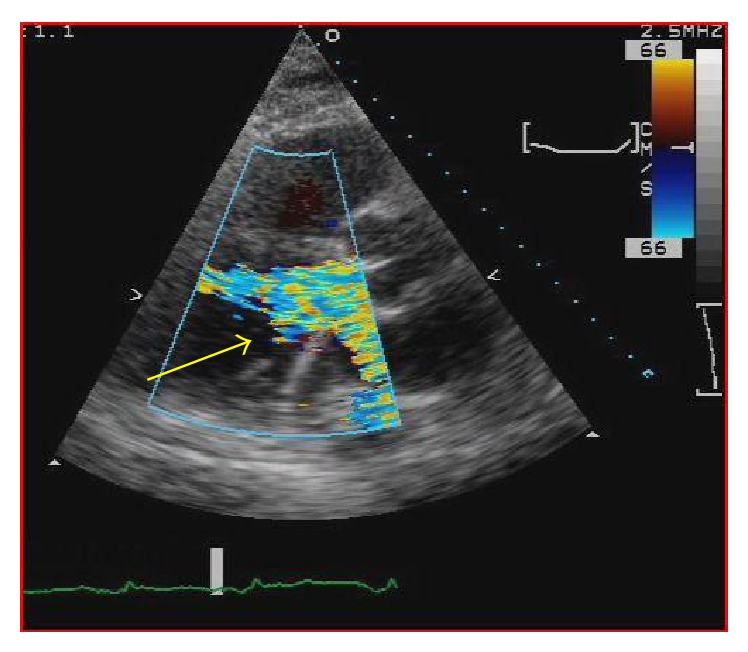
Parasternal long axis view with color flow showing the turbulence of the Impella located subvalvular (yellow arrow).
